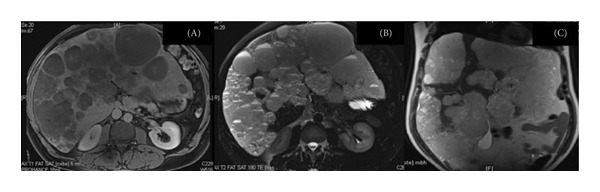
Magnetic resonance imaging (MRI) of the abdomen demonstrating massive hepatomegaly measuring 31 cm (previously 27 cm) with diffuse cystic involvement. (A) Axial T1‐weighted image demonstrating innumerable predominantly hypointense hepatic cysts, several with intrinsic T1 hyperintensity consistent with hemorrhagic or proteinaceous content. (B) Axial T2‐weighted image demonstrating markedly hyperintense cystic lesions with fluid–fluid levels and internal complexity. (C) Coronal T2‐weighted image demonstrating severe craniocaudal hepatomegaly with diffuse cystic replacement, a dominant left hepatic lobe cyst measuring 9.5 × 8.7 cm, and associated narrowing of the left portal vein, distortion of the left and middle hepatic veins, and mild inferior vena cava compression.
